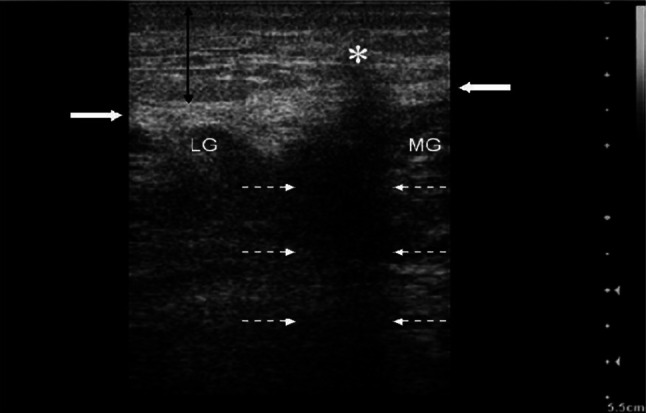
Bedside ultrasonography on the left calf with necrotizing fasciitis. Anechoic area in superficial soft tissue (*), caused by fat necrosis and suppurative infiltration, with posterior shadowing (broken opposing white arrows), which seems to break the underlying fascia. Subcutaneous tissue appears thick, and hyperechoic due to diffuse inflammatory infiltrate (vertical arrow)—source: Testa et al. with permission
